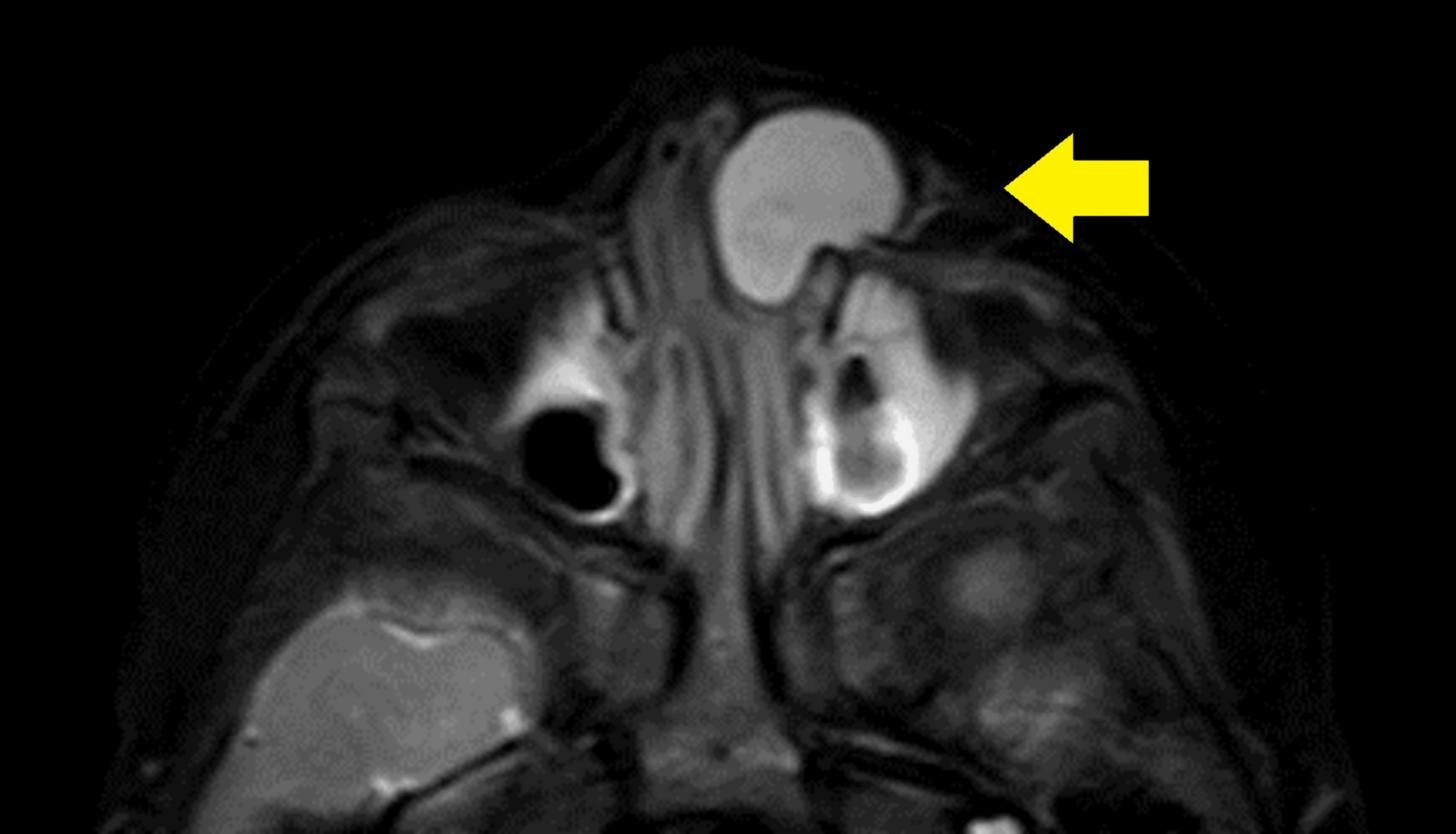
T2-weighted short TI inversion recovery image showing a cystic lesion with high signal intensity.
